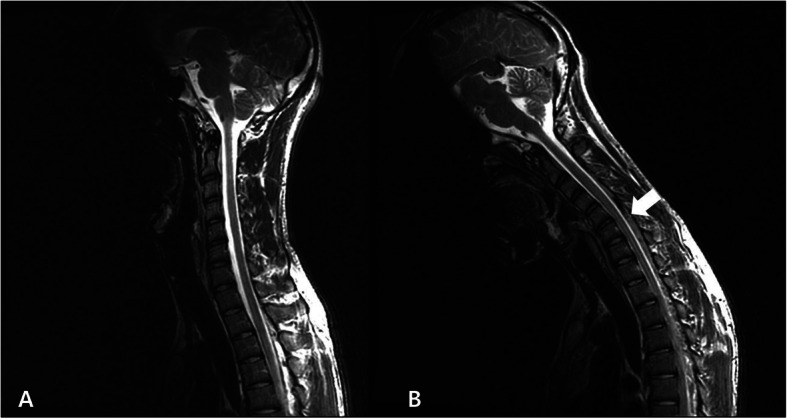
Cervical magnetic resonance images captured with the patient in the supine position (a) were unremarkable, while when the patient was in the flexed position, anterior effacement of the posterior dural sac was observed with venous engorgement at the C6–8 levels (b)
